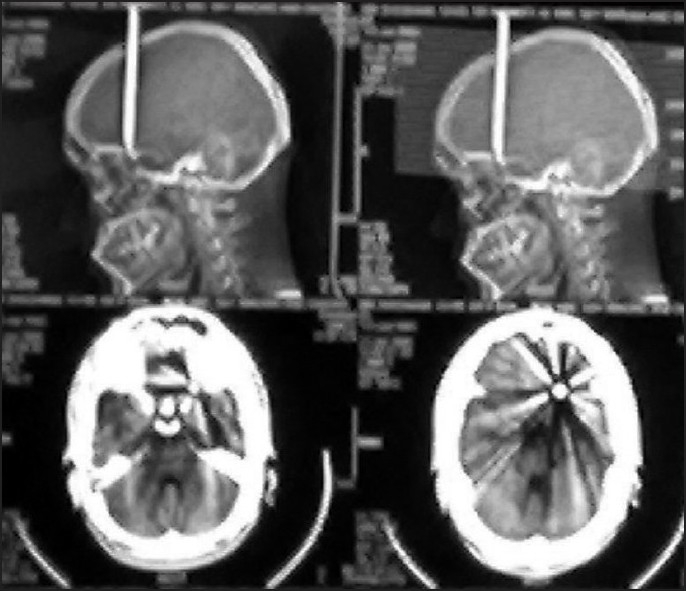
CT scan head-showing craniocerebral injury
